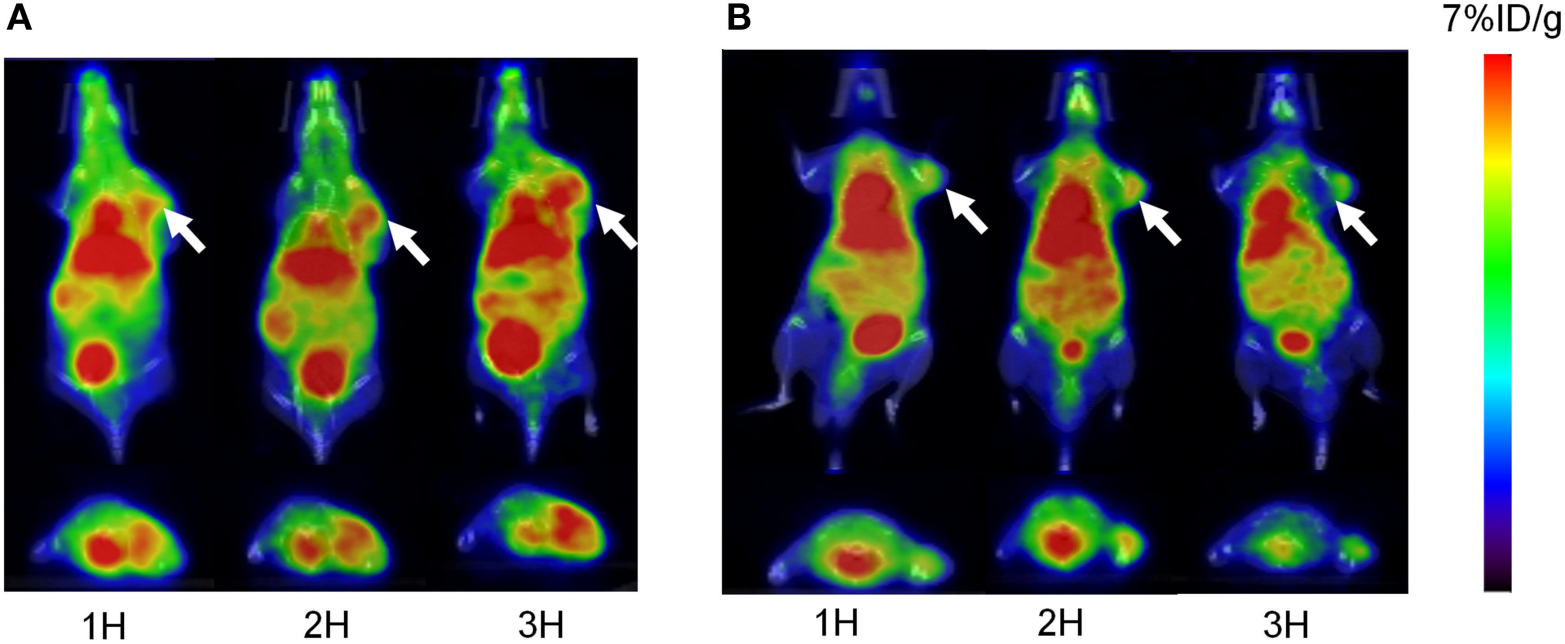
The overlaying of the PET and CT images of H22 tumors acquired at 1, 2, and 3 h after the intravenous injection of (A)
